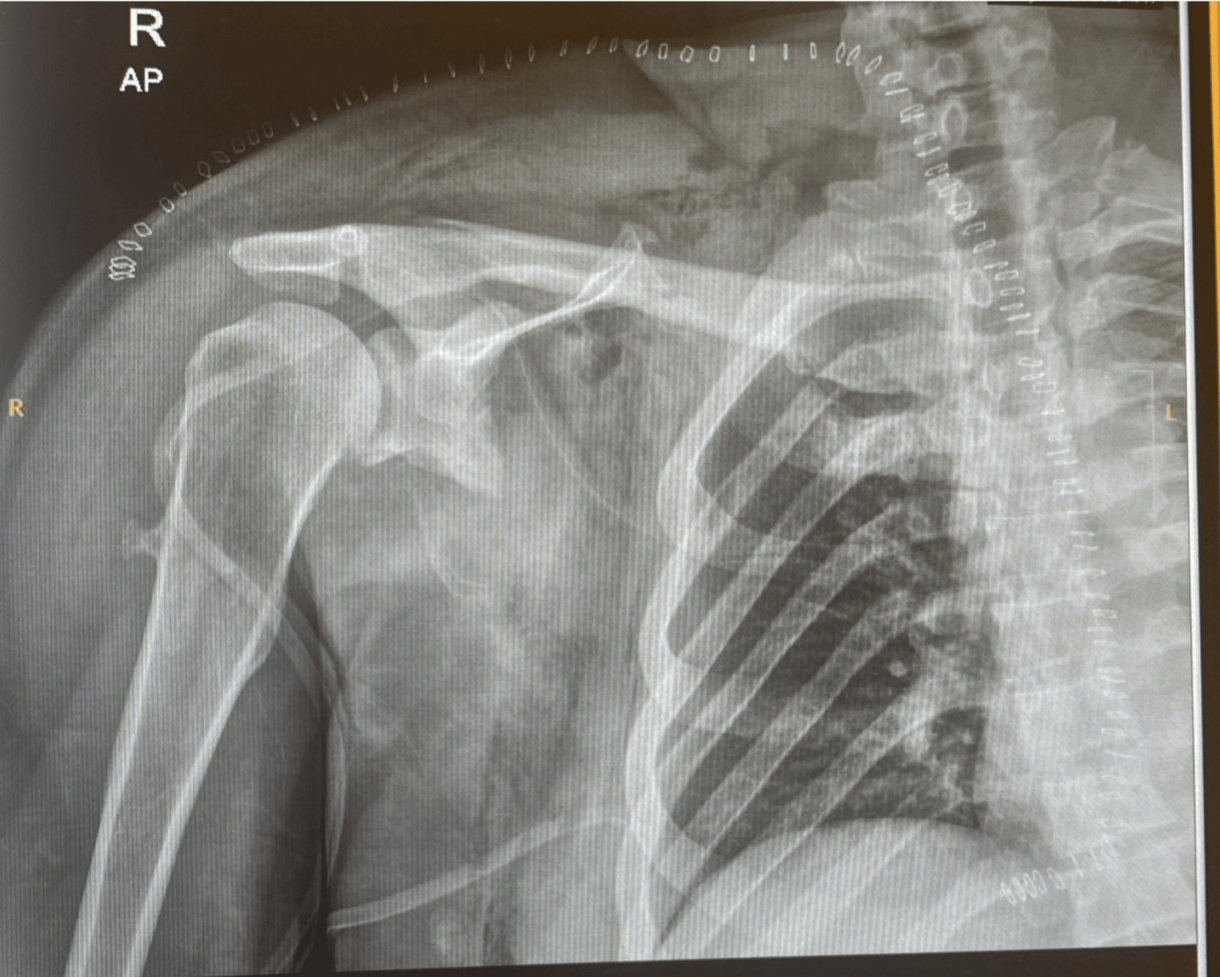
Postoperative X-ray of the right shoulder.
